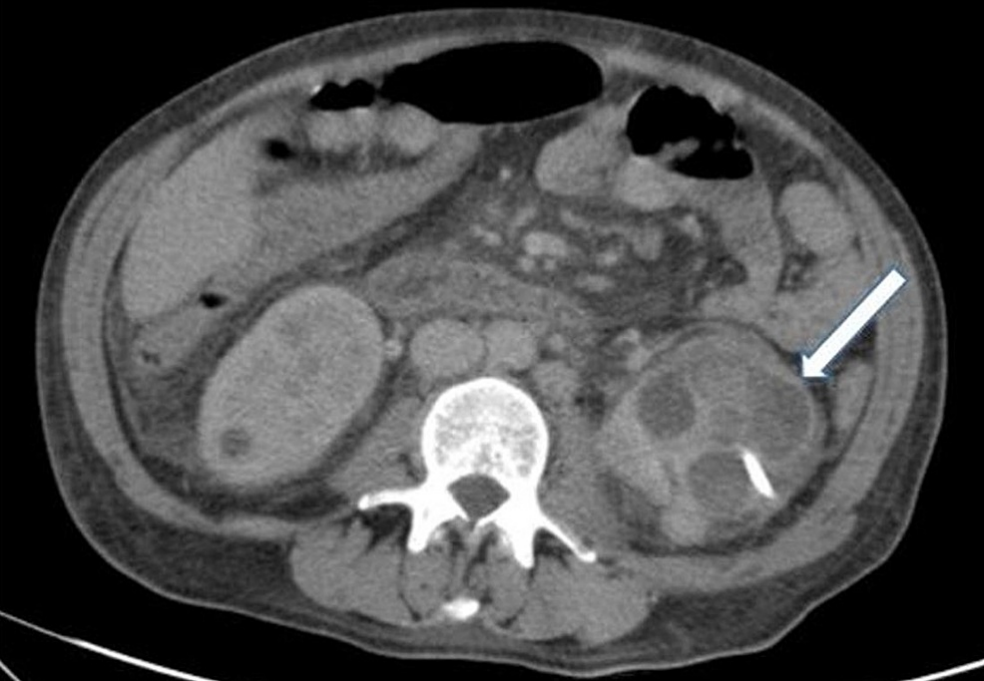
Abdominopelvic CT scan showing the reduction in the right abscess and significant increase on the left, with the presence of new abscesses (white arrow) CT: computed tomography
